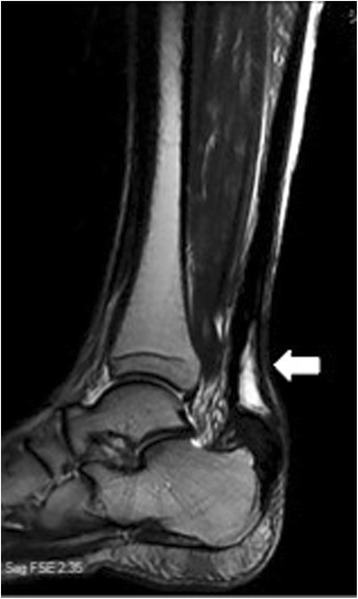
Preoperative magnetic resonance imaging. Parts of the Achilles tendon remained intact, but the tendon was extended (*arrow*)
